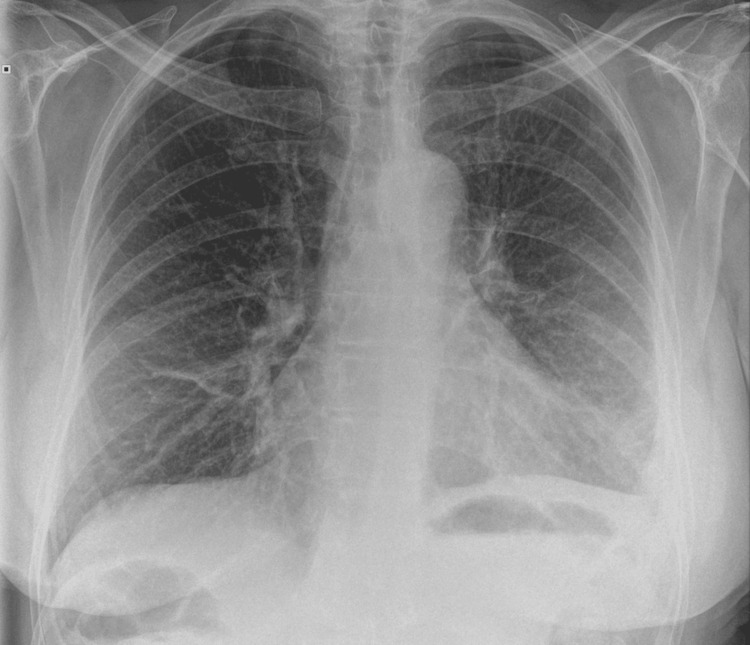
Chest radiograph demonstrating left-sided basal consolidation.
